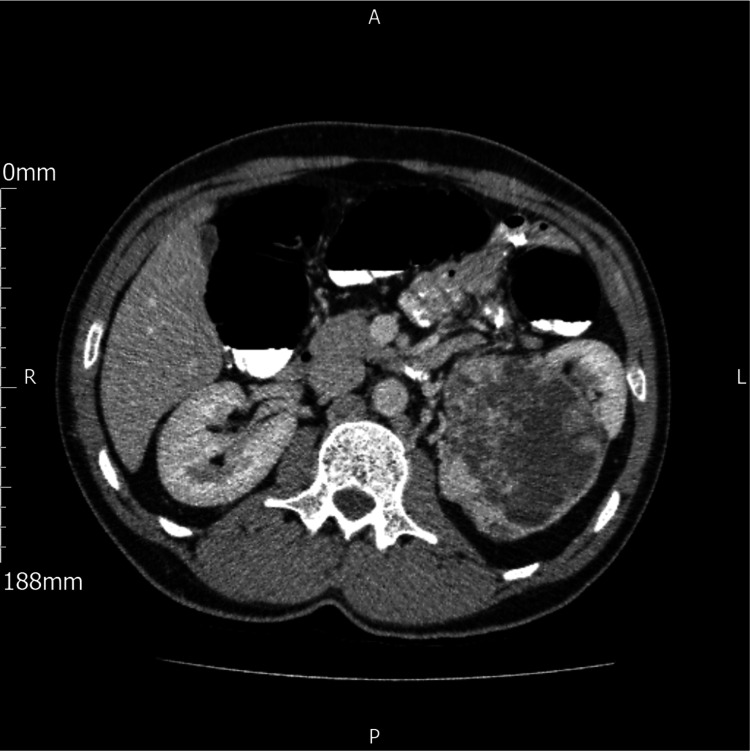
Cross-sectional image from CT colonography. A solid mass can be visualised in the left kidney.
